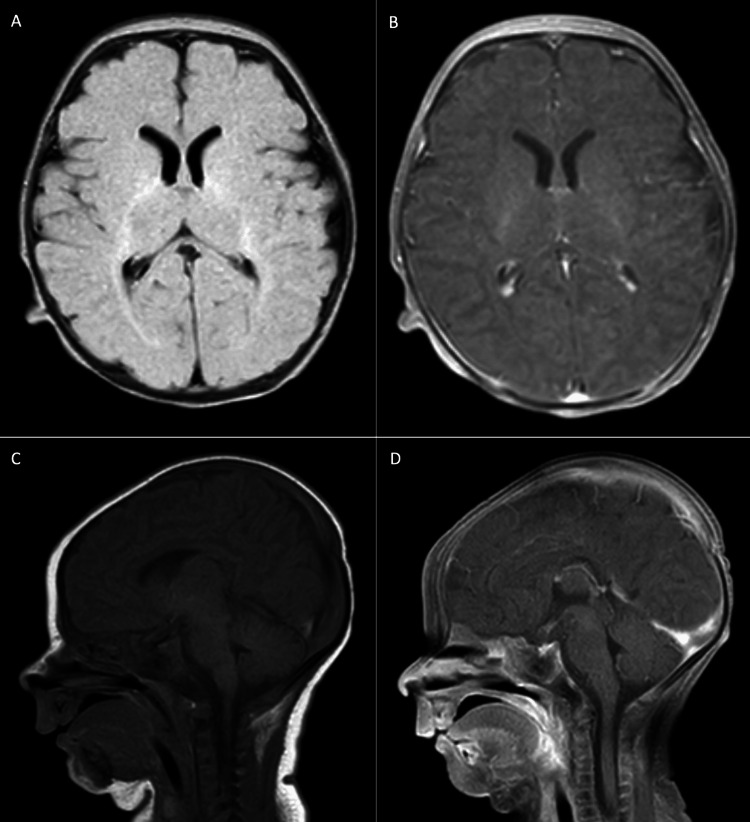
Normal MRI brain with and without contrast. A) Axial view of brain without contrast. B) Axial view of brain with contrast. C) Sagittal view of brain without contrast. D) Sagittal view of brain with contrast. MRI: magnetic resonance imaging
